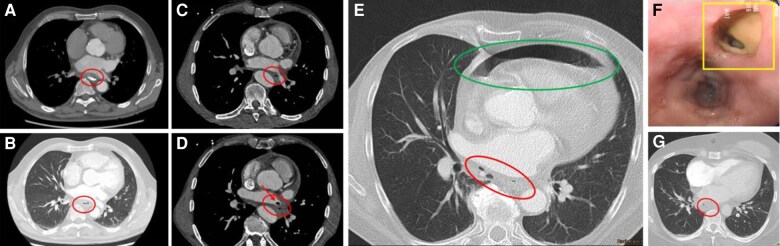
Characteristic CT (*A*–*E*, *G*) and endoscopy (*F*) findings in patients with *A* + *B*) oesophageal perforation, *C* + *D*) atrio-oesophageal fistula, *E*) oesophago-pericardial fistula, and *F* + *G*) perforating oesophageal ulcer. *A*) Air in mediastinum (red circle) with exit of water-soluble oral contrast into the mediastinum, *B*) i.v. contrast and documentation of air in mediastinum (red circle), *C* and *D*) air in mediastinum (red circle) and air and thrombus in the left atrium (arrow), *E*) i.v. contrast and identification of air in mediastinum (red circle) and pericardium (green circle), *F*) endoscopic finding 5 days after PV isolation with perforated oesophageal ulcer (yellow square), and *G*) corresponding CT with air in mediastinum (red circle). CT, computed tomography; PV, pulmonary vein.
